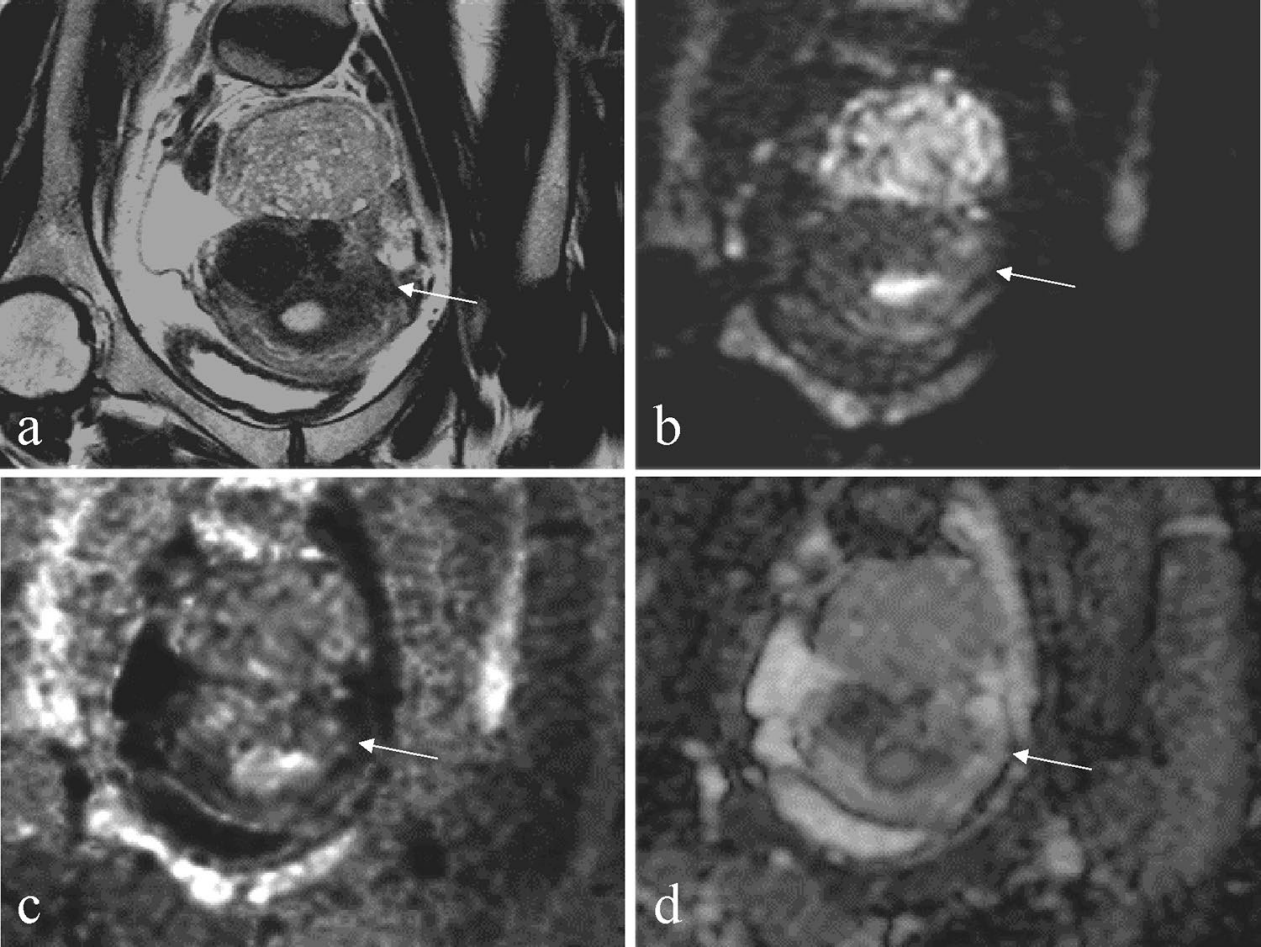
A 42-year-old woman with cellular leiomyoma without abnormal vaginal bleeding. a The coronal T2-weighted image reveals a tumor locates mainly in the subserosal location. The tumor shows a higher signal intensity than the myometrium on a T2-weighted image. b On a coronal diffusion-weighted image with a *b*-value of 1000 s/mm^2^, the lesion shows a higher signal than the myometrium (thin arrow). However, c the signal was equal to that of the myometrium on a coronal computed diffusion-weighted image with a *b*-value of 2000s/mm^2^. d Using the coronal apparent diffusion coefficient map, the lesion is determined to have a mean apparent diffusion coefficient value of 1.65 × 10^−3^ mm^2^/s
